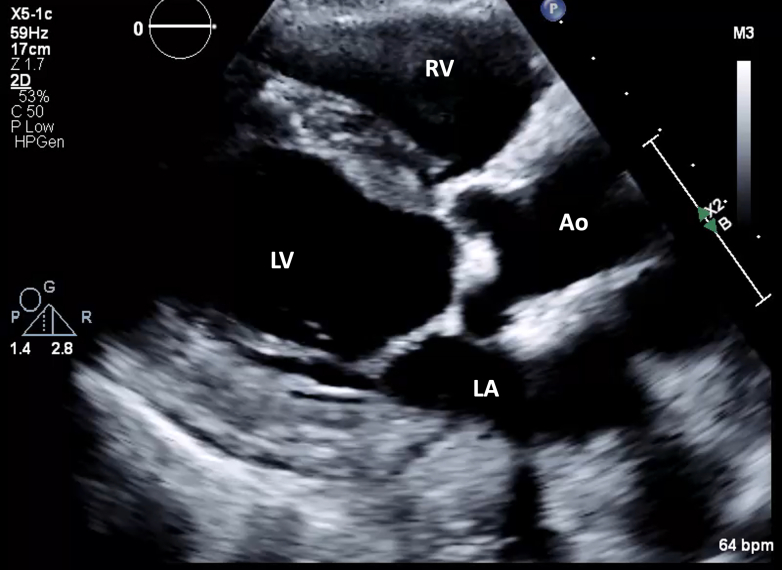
Parasternal Long-Axis View Using your transthoracic echocardiogram transducer, start in the parasternal long-axis view to assess LV ejection fraction and wall thickness. Ao = aorta; LA = left atrium; LV = left ventricular; RV = right ventricular.
